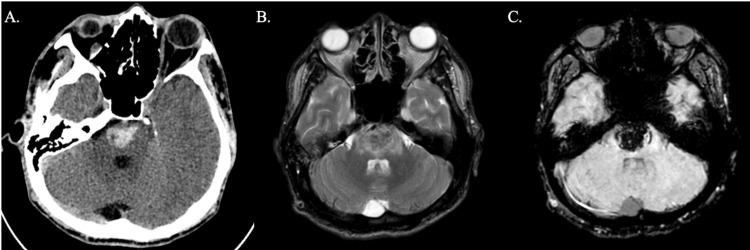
(A) Plain cranial CT scan axial view demonstrating an acute pontine hemorrhage measuring 1.7 × 1.9 × 1.7 cm amounting to approximately 2.8 cc. Cranial MRI with contrast (B) axial view T2 and (C) axial view GRE sequences demonstrating a subacute pontine hemorrhage.
